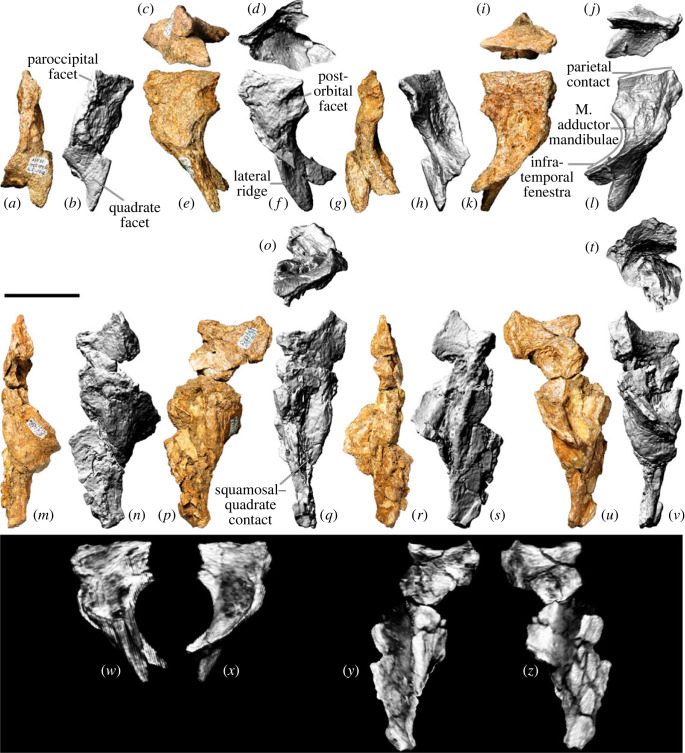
Diamantinasaurus matildae* referred squamosals (AODF 0906). (*a–l*) Right squamosal in (*a,b*) posterior, (*c,d*) ventral, (*e,f*) lateral, (*g*,*h*) anterior, (*i,j*) dorsal and (*k,l*) medial views. (*a*), (*c*), (*e*), (*g*), (*i*) and (*k*) are photographs, (*b*), (*d*), (*f*), (*h*), (*j*) and (*l*) are three-dimensional models derived from surface scans. (*m–v*) Left squamosal (with attached left quadrate fragment) in (*m*,*n*) posterior, (*o*) ventral, (*p*,*q*) lateral, (*r*,*s*) anterior, (*t*) dorsal and (*u*,*v*) medial views. (*m*), (*p*), (*r*) and (*u*) are photographs, (*n–o*), (*q*), (*s–t*) and (*v*) are three-dimensional models derived from surface scans. (*w,x*) Three-dimensional digital models (derived from medical CT data) of the right squamosal in (*w*) lateral and (*x*) medial views. (*y,z*) Three-dimensional digital models (derived from medical CT data) of the left squamosal in (*y*) lateral and (*z*) medial views. Scale bar = 50 mm.
